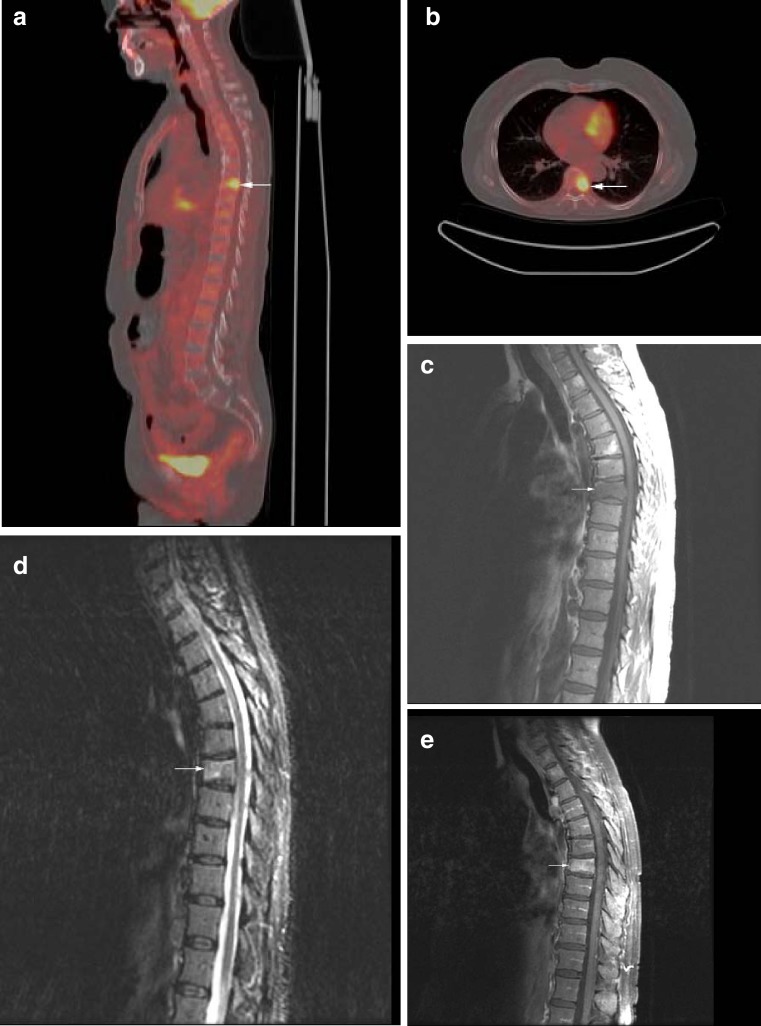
a Malignant compression fracture from metastatic colon cancer. Sagittal fused FDG-PET/CT image demonstrates intense radiotracer uptake in the T7 compression fracture (SUV = 6.3;* arrow*). b Axial fused FDG-PET/CT image demonstrates focal increased radiotracer uptake in the T7 vertebral body (*arrow*). c Sagittal T1-weighted MRI demonstrates compression fracture of T7 with low signal marrow infiltration (*arrow*). d Sagittal STIR image demonstrates mild hyperintensity of the T7 vertebral compression fracture (*arrow*). e Sagittal fat-suppressed T1-weighted MRI following the administration of gadolinium demonstrates diffuse enhancement of the T7 compression fracture (*arrow*). Malignancy was indeterminate on MRI
